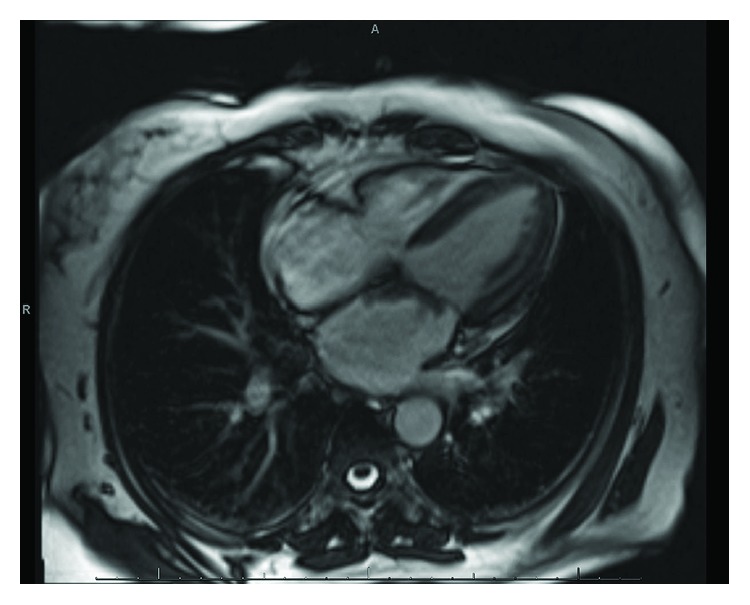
Cardiac MRI first-pass perfusion imaging showing mass originating on the atrial septum and extending along the atrial aspect of the anterior mitral valve leaflet.
